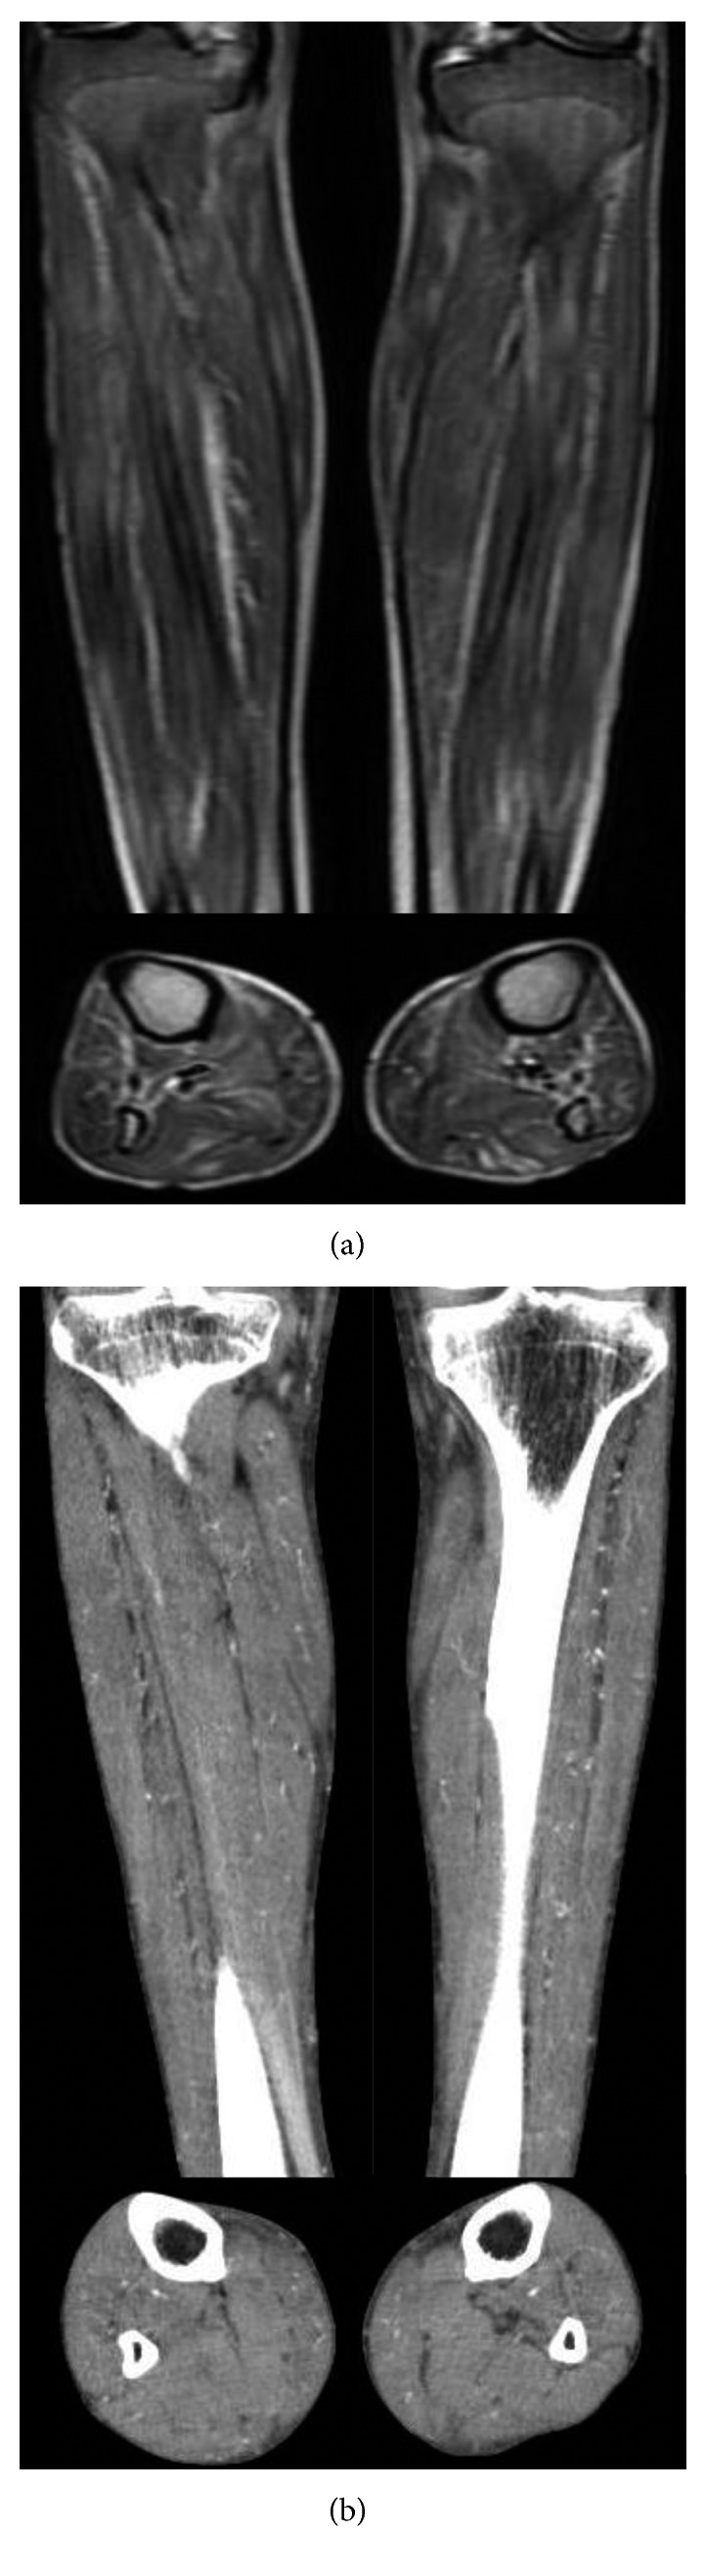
MRI/CT image of lower legs. (a) STIR (short tau inversion recovery) magnetic resonance image showing high-signal intensity areas in the lower leg muscles. These lesions had high DWI signals and normal ADC values. The muscle structure was intact. (b) Noncontrast enhanced computed tomography showing high density areas along the vessels.
